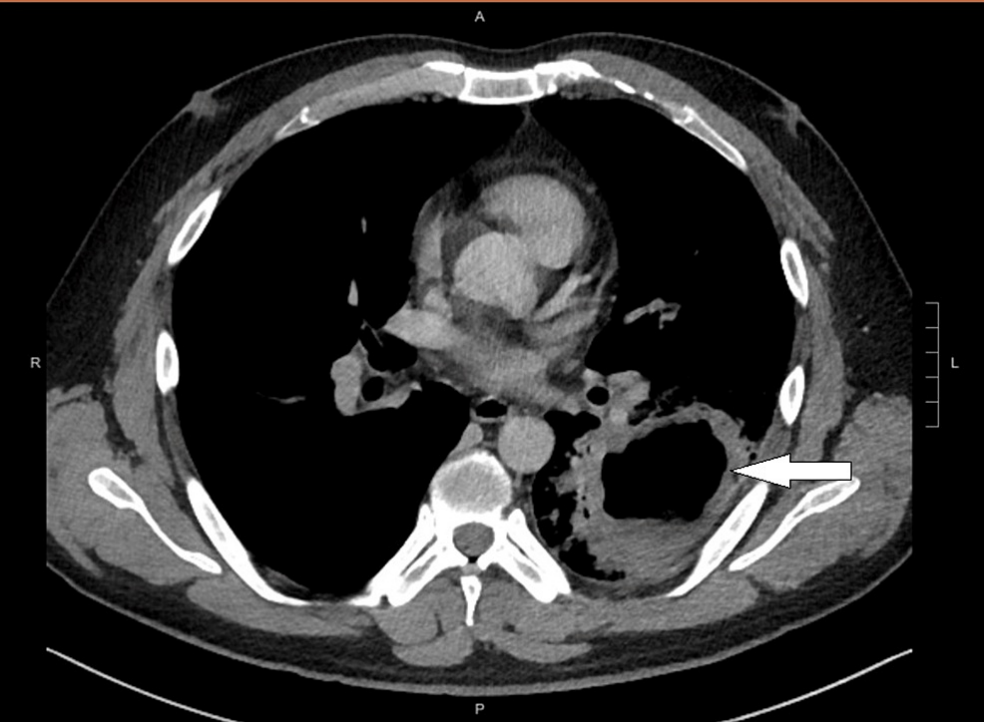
Contrast-enhanced CT image of the patient before the CA-MRSA treatment The white arrow points toward an 8.3 × 6.2 × 8.7 cm lung abscess in the left lower lobe. CA-MRSA, community-acquired methicillin-resistant *Staphylococcus aureus*
